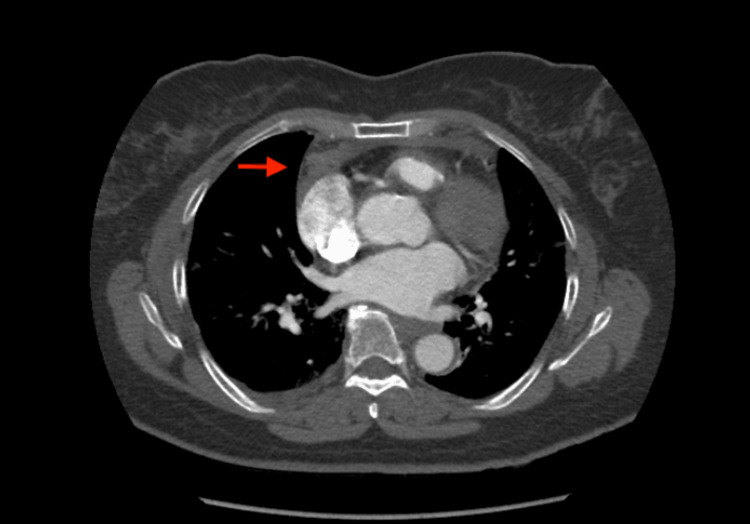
Axial view CT chest. Red arrow indicating the anterior mediastinal hematoma.
